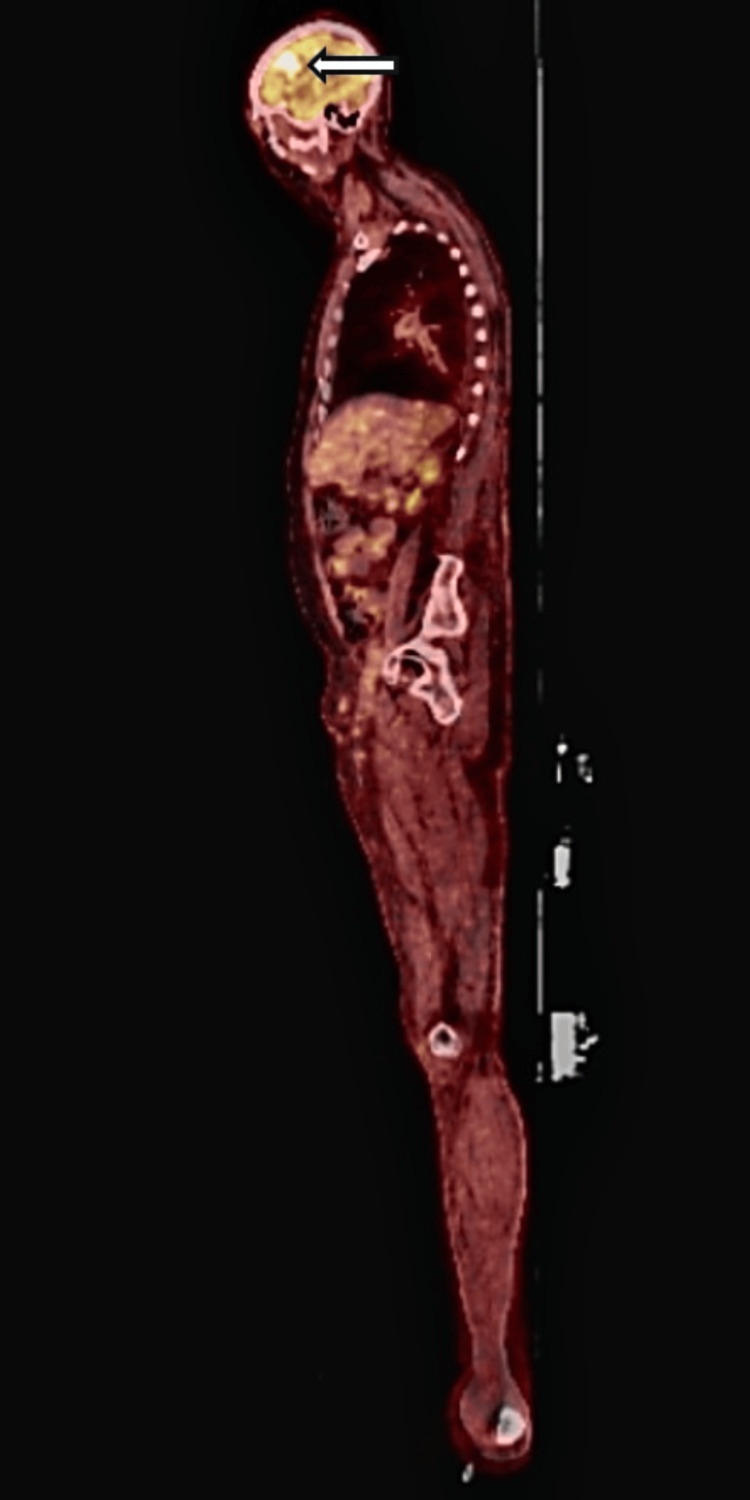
PET/CT showing an FDG-avid lesion in the right frontal cortex approximately 60 minutes after intravenous administration of 10.56 mCi of FDG. No metastasis was present. FDG: F18-fluorodeoxyglucose
